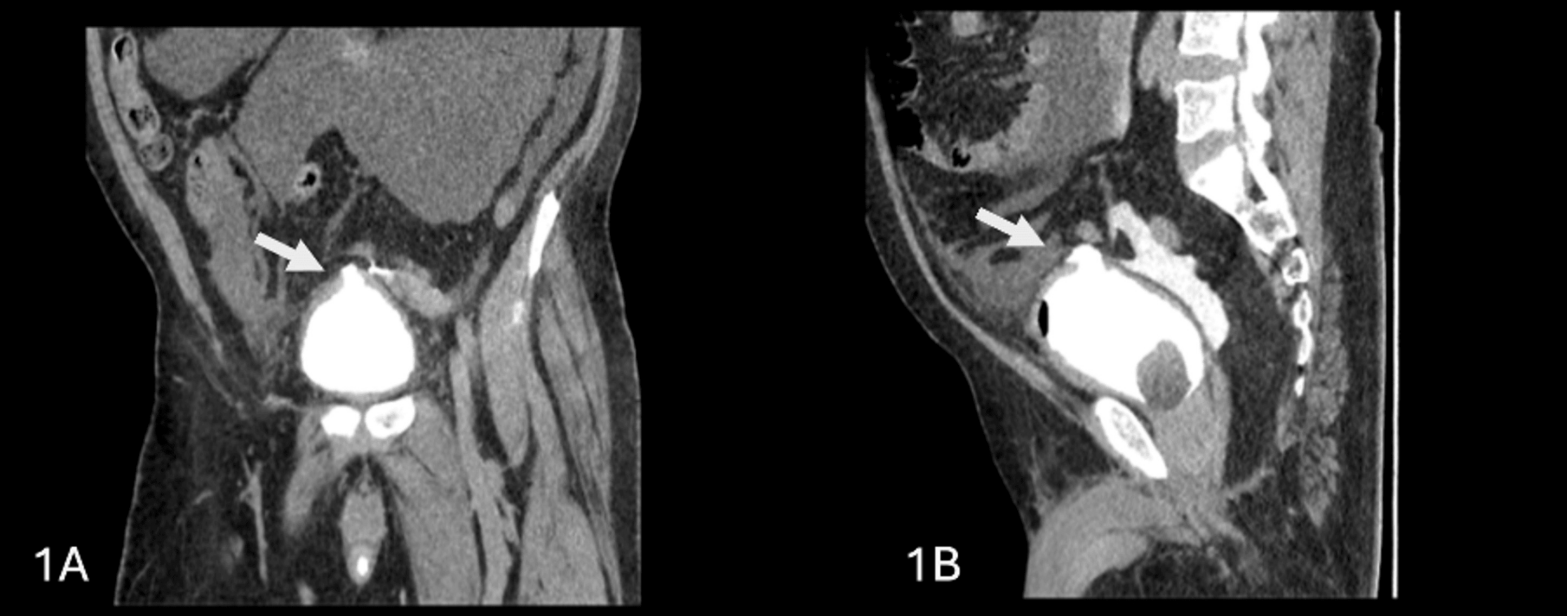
(A) Coronal view of computed tomography cystogram showing superior bladder wall defect with extravasation of intravesical contrast. (B) Sagittal view.
